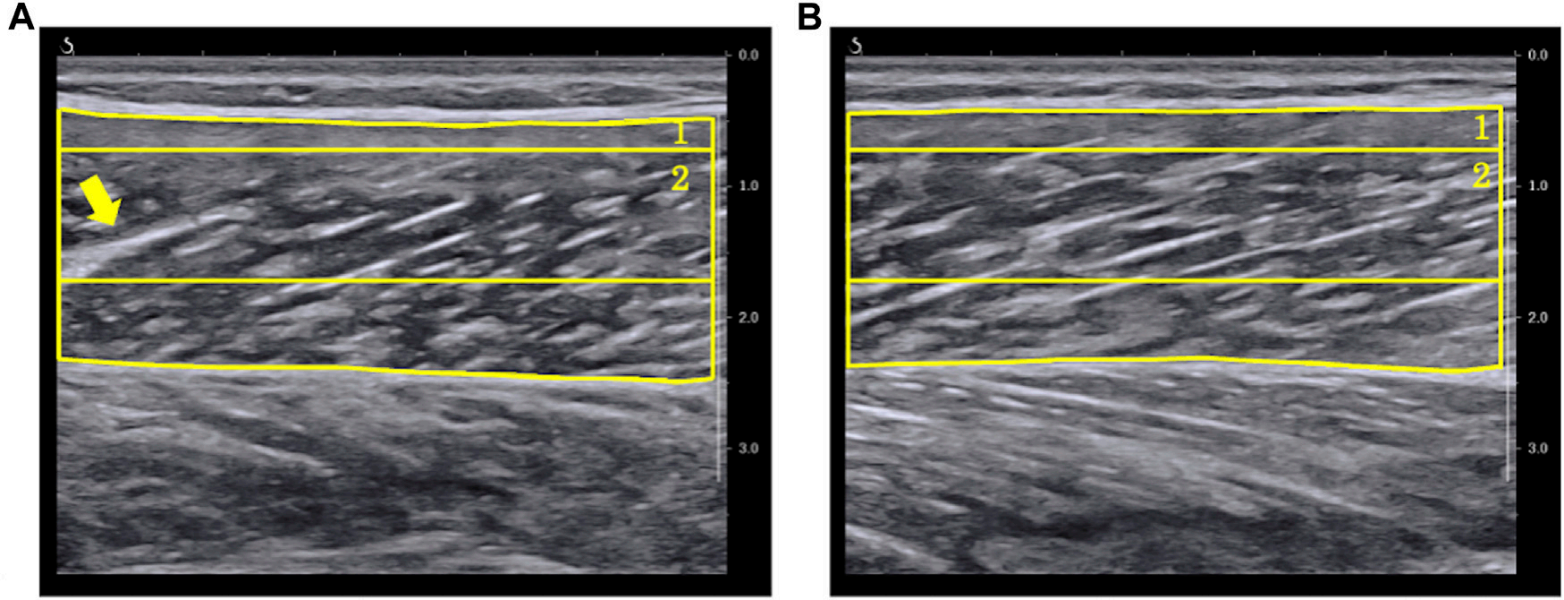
Ultrasound B-mode images with the ankle in (A) 20° plantar-flexion and (B) 20° dorsiflexion for the participant who showed minimum *r*-value between echo intensity and ankle angle. In the image at 20° ankle plantar-flexion, the arrow signifies a fiber (displayed in white) that presents like an intramuscular tendon. (1) A region of interest (RoI) which included as much of the muscle as possible while avoiding fasciae and bone (maximum RoI). (2) A region of interest whose size and depth were identical for all images of all participants (rectangular RoI).
